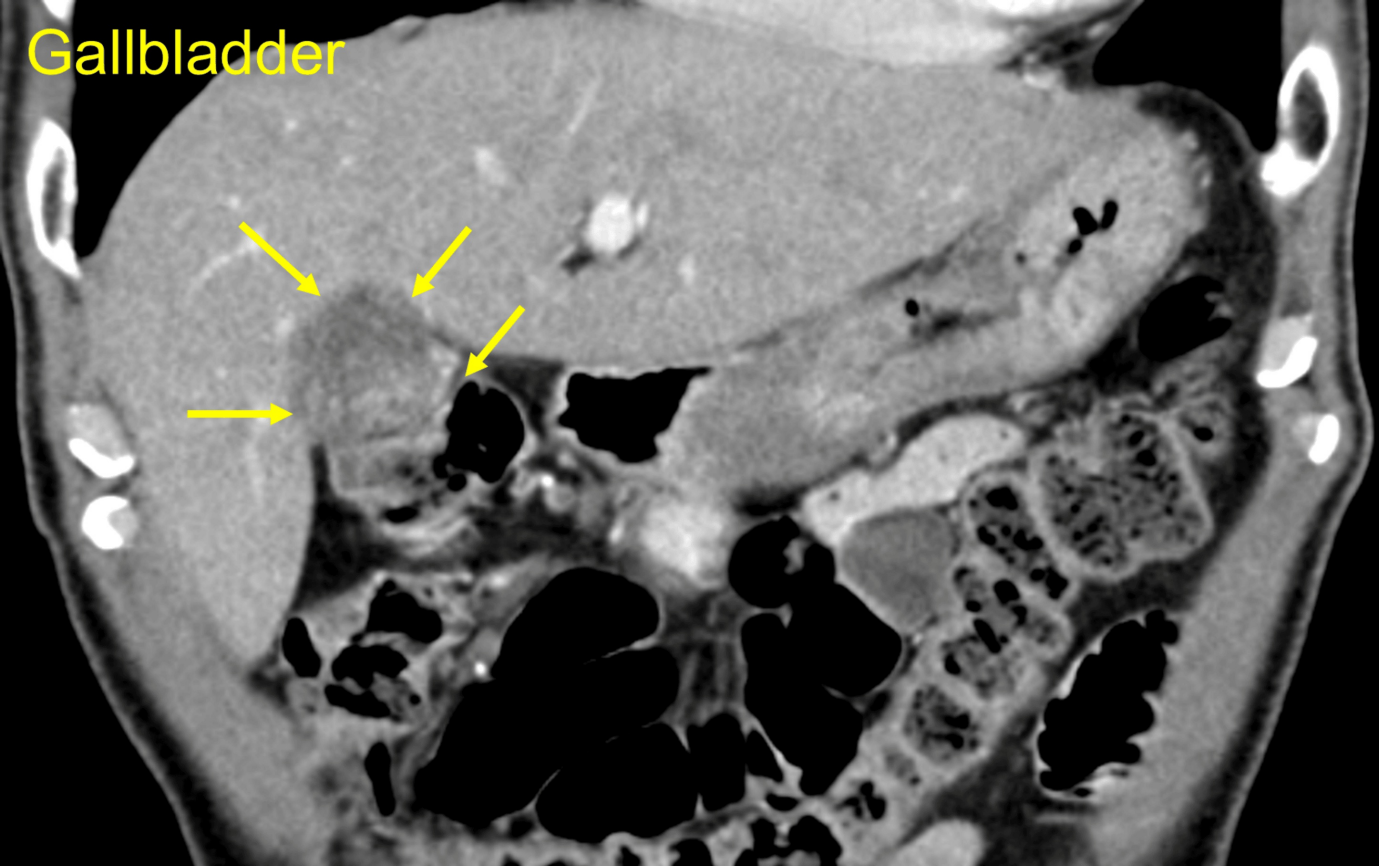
Abdominal computed tomography scan on admission Yellow arrows indicate the irregularly thickened gallbladder with a tumor filling the lumen. The border between the liver and gallbladder is unclear. The gallbladder seems to adhere to the transverse colon, suggesting gallbladder carcinoma invasion
